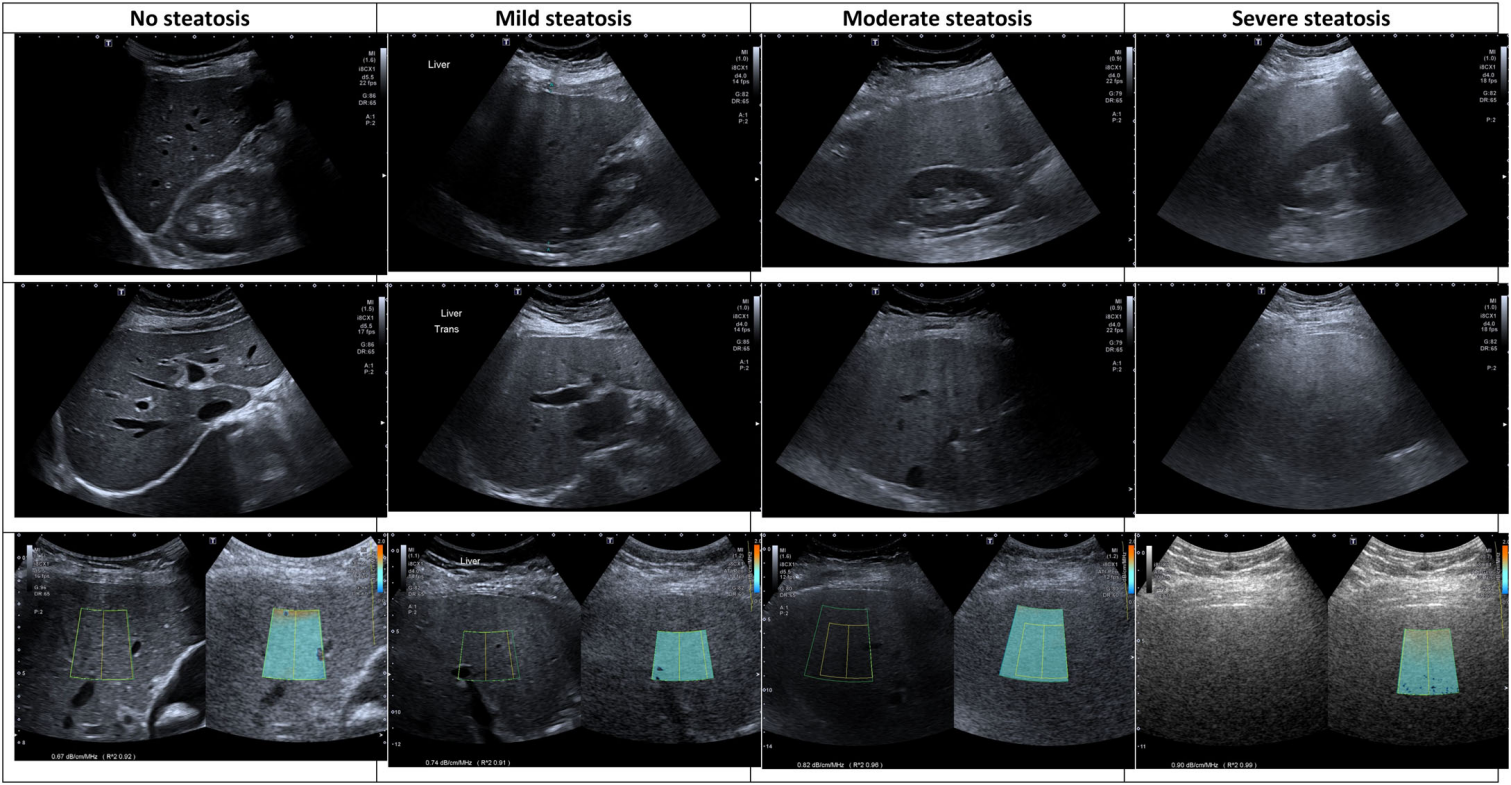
Selected ultrasound images from study patients with no steatosis, mild steatosis, moderate steatosis and severe steatosis (left to right) based on the Radiologist's overall impression. Images in the top row demonstrate liver–kidney contrast, and images in the middle row highlight vessel wall blurring, posterior beam attenuation and diaphragmatic definition. Images in the bottom row demonstrate a single attenuation imaging measurement for each patient, in these examples: 0.67, 0.74, 0.82 and 0.90 dB/cm/MHz respectively. Note that the *R*
